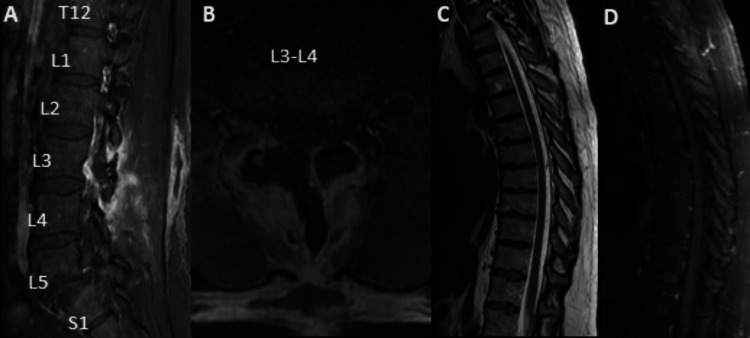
Post-operative magnetic resonance imaging of the lumbar spine (sagittal and axial views). Sagittal (A) and axial (B) T1WI of the lumbar spine show post-operative imaging findings, with complete resection of the right foraminal hemangioblastoma at L3-L4 level. Sagittal T2WI (C) and sagittal T1WI fat-saturated (D) of the thoracic spine depict the absence of flow voids in the dorsal aspect of the spinal cord.
